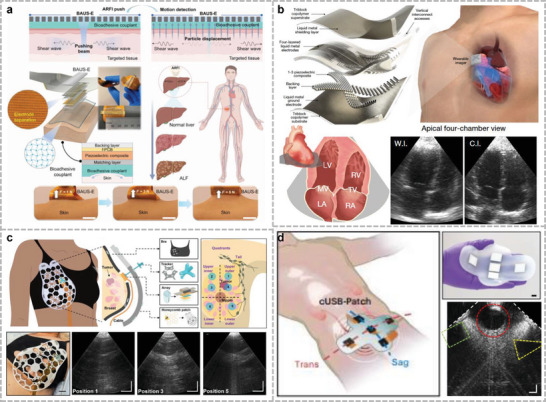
Continuous organ imaging with wearable ultrasonic transducer. a) Wearable bio‐adhesive ultrasound elastography (BAUS‐E) generates acoustic radiation force impulses (ARFI) to induce shear waves for elastography measurements, which can be used to observe stiffness changes in the human liver. Reproduced with permission.^[^
